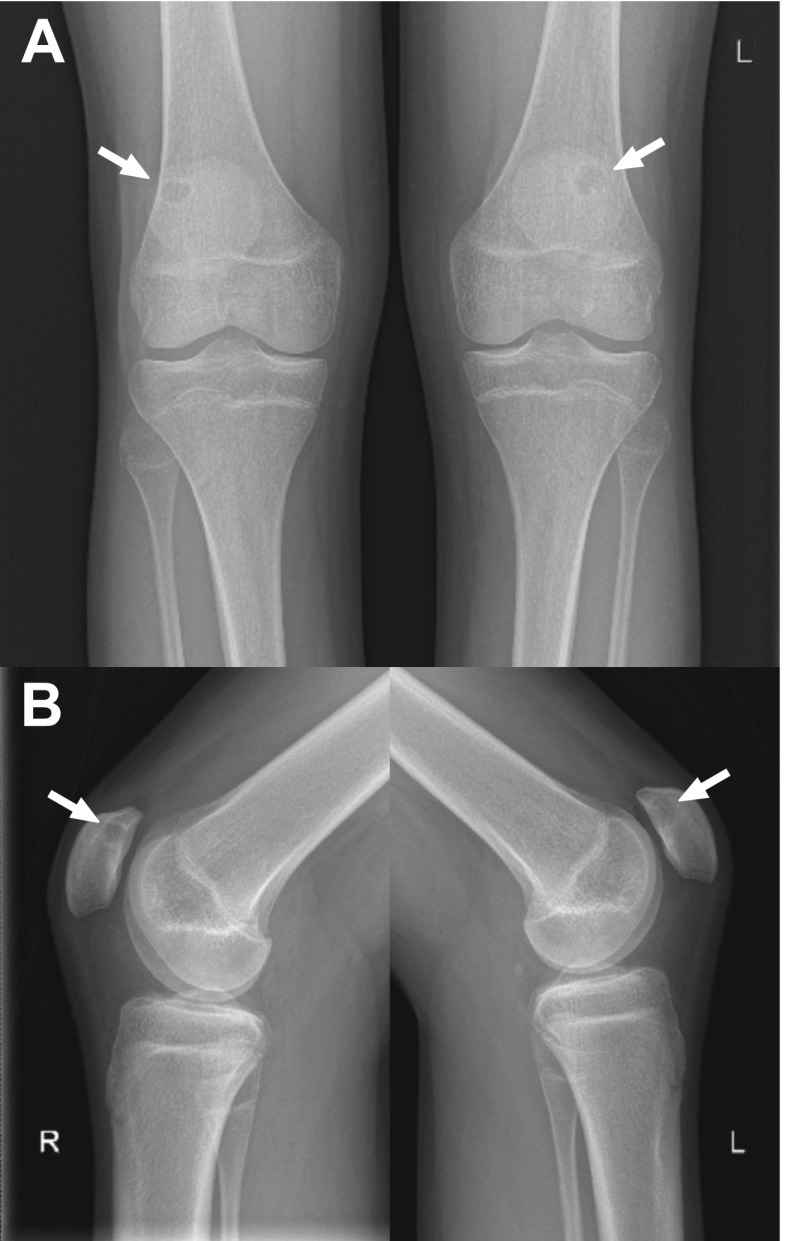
Eight-month follow-up frontal (a) and lateral (b) radiographs of both knees still demonstrate the dorsal patellar defects (arrows), without any obvious changes compared to 8 months earlier (Fig. 1). However, the patient was almost symptom-free at the time of this radiographic examination
